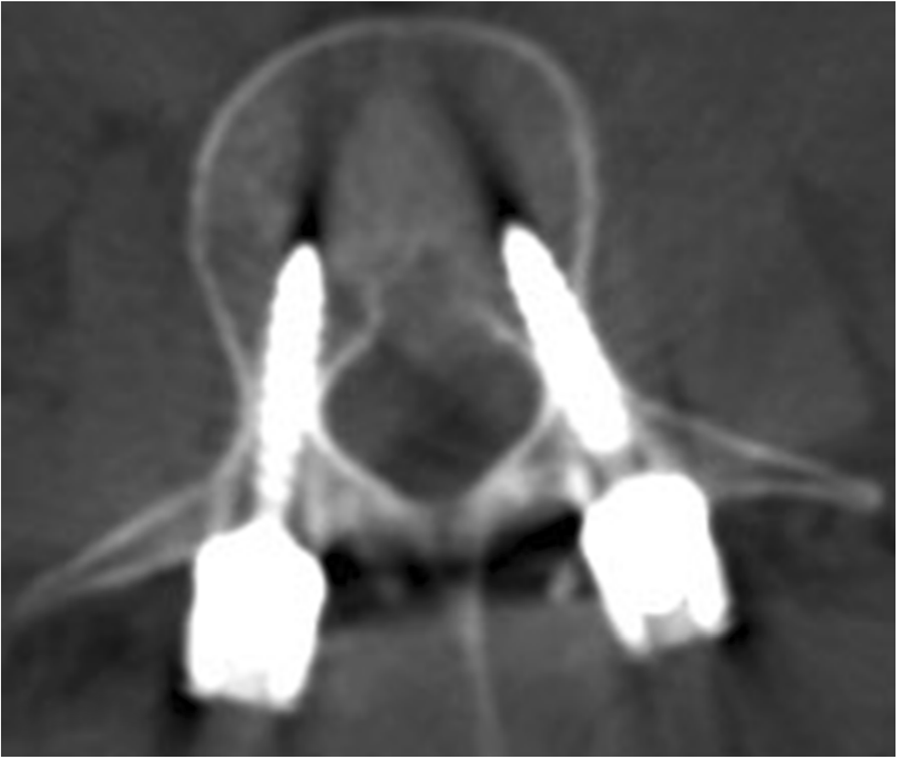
CT scan demonstrates reduction of canal encroachment after operation
